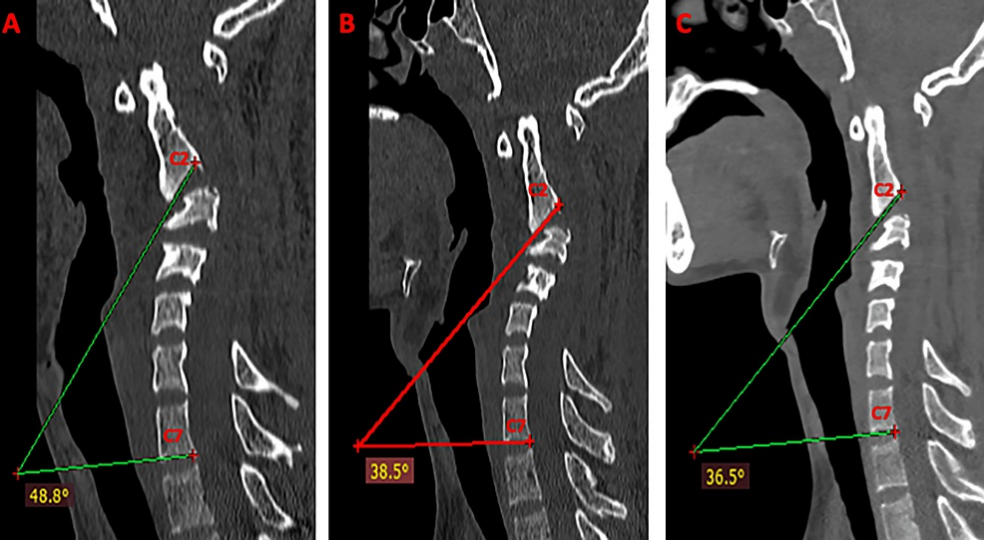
CT sagittal view showing a gradual reduction in the global kyphosis/Cobb’s angle with a gradual increment in the traction weights. (A) 3 Kg; global Cobb’s angle of 48.8 degrees. (B) 5 kg; global Cobb’s angle of 38.5 degrees. (C) 8 kg; global Cobb’s angle of 36.5 degrees.
